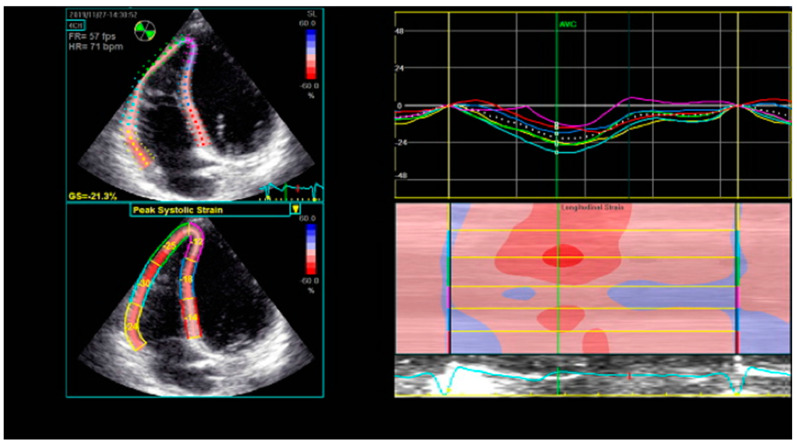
Six-segment model of longitudinal RV strain using STE. RV—right ventricle; STE—speckle-tracking echocardiography.
